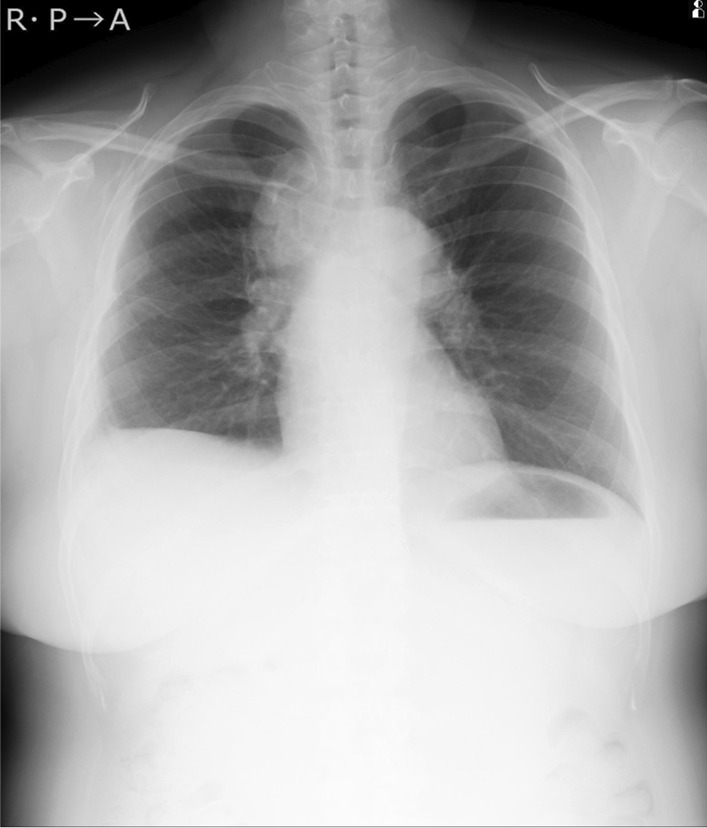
Chest X-ray at 2 weeks after administration of steroid
